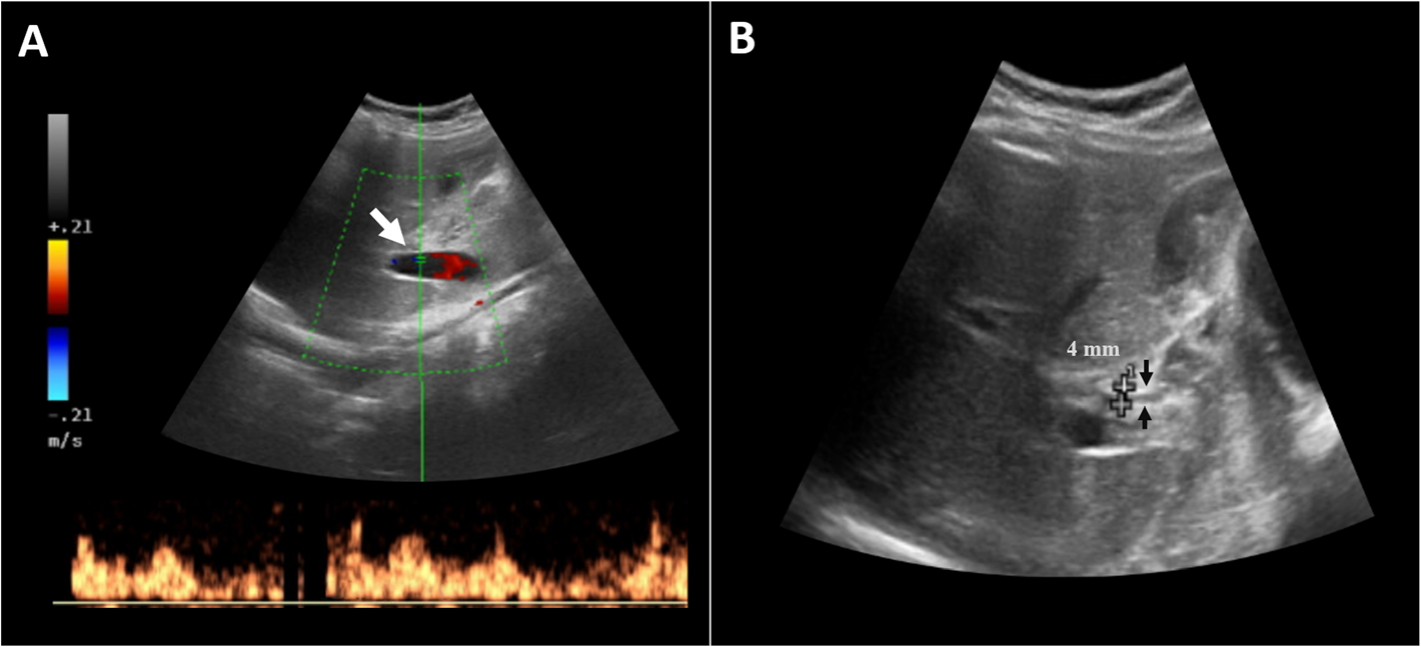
Abdominal ultrasound image showing normal hepatic echogenicity with hepatopetal flow in portal vein (arrow in a) and non-dilated common bile duct (arrows in b)
